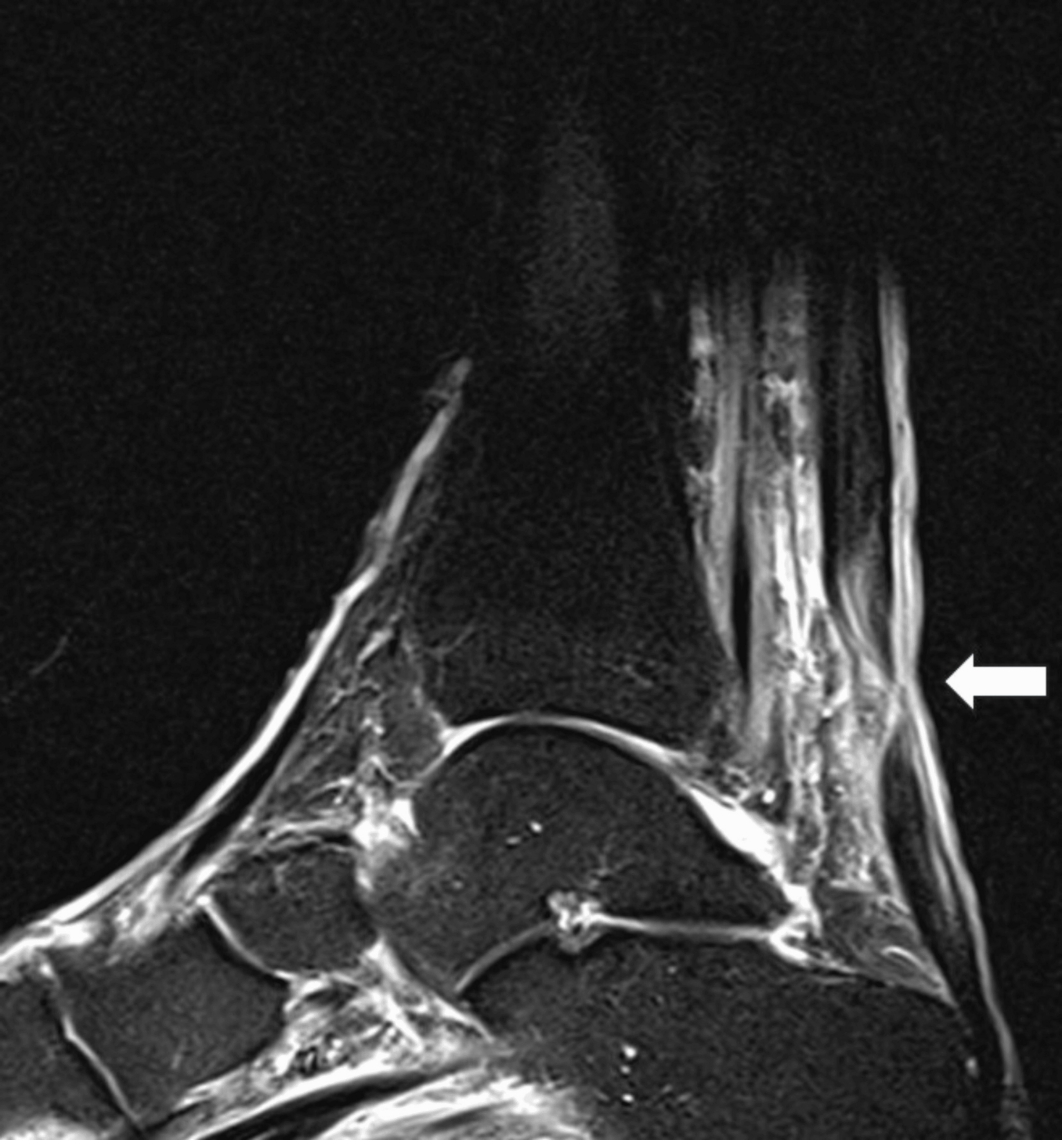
Achilles tendon rupture diagnosis is confirmed with an ankle MRI. Achilles tendon rupture is noted 4.4 cm proximal to the calcaneal insertion (arrow). The tendon stumps present minimal retraction. Increased intratendinous signal is indicative of some degree of Achilles noninsertional tendinopathy in T2 sequence. MRI: Magnetic Resonance Imaging
